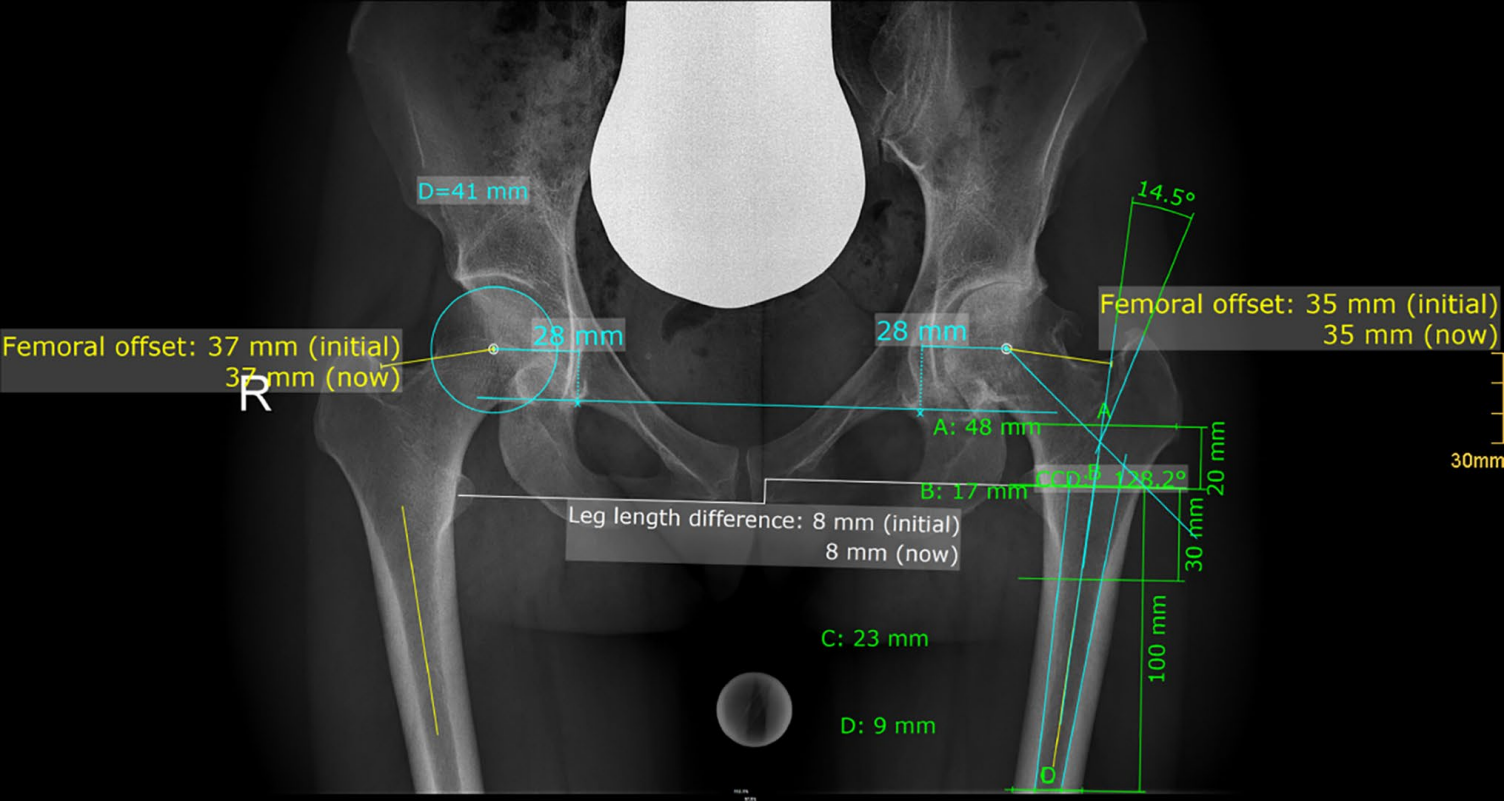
Preoperative measurements: HO, FO, AO, LLD (all bilaterally), CTA, CCD angle, Canal Flare Index, Cortical Index, canal to calcar ratio (all unilaterally)
